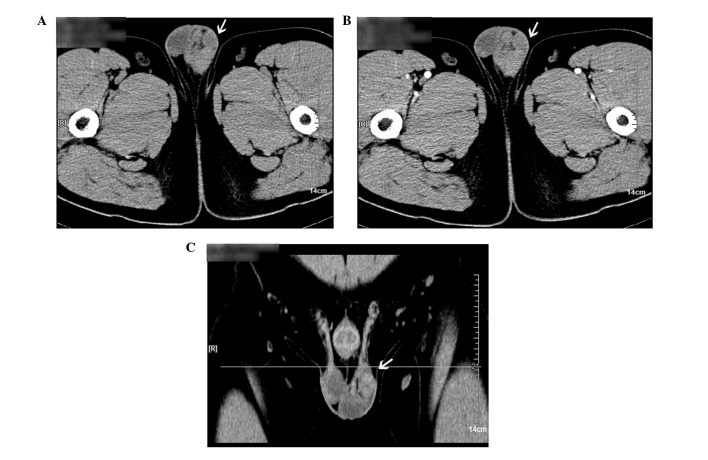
CT images of an SFT in the spermatic cord: (A) Unenhanced CT scan; (B) enhanced CT scan (the mass density is enhanced slightly); and (C) coronal section of the enhanced CT scan. SFT, solitary fibrous tumor; CT, computed tomography.
